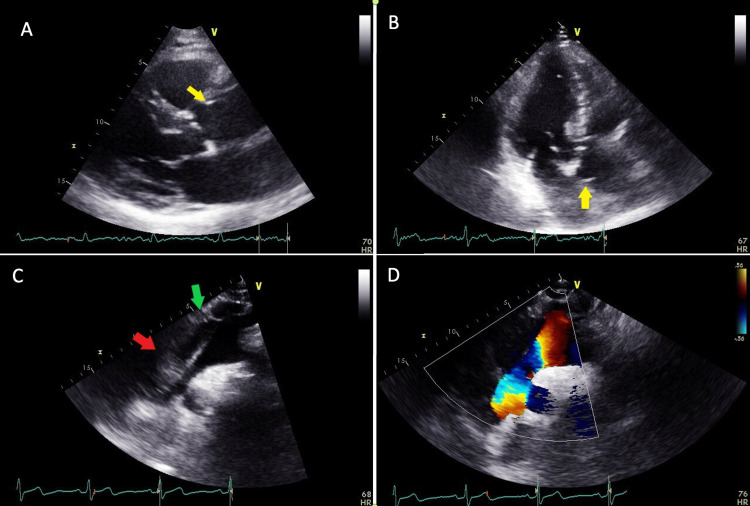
Echocardiogram images of the ascending type A dissection. Panel A: Parasternal long-axis view showing the dissection flap (yellow arrow) and dilated ascending aorta. Panel B: Apical three-chamber view showing the dissection flap (yellow arrow). Panel C: Suprasternal view showing the false lumen of the dissection (green arrow). Panel D: Suprasternal view showing no flow on color Doppler in the false lumen.
